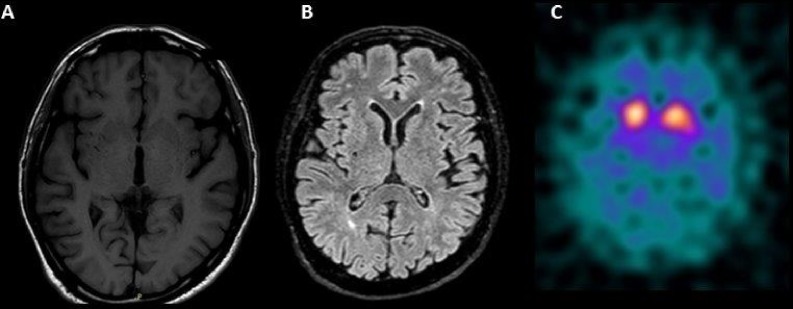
(A) Bilateral hypointensities in T1 in the striatum, more prominent on the right side and (B) rare small subcortical white matters hyperintensities on T2 (mainly periventricular and frontal) on Magnetic Resonance Imaging of the brain. (C) Single Photon Emission Computerized Tomography of the Dopamine Transporter (DAT) with 123I-ioflupane showing a significant low uptake of DAT, mostly in the right striatum.
